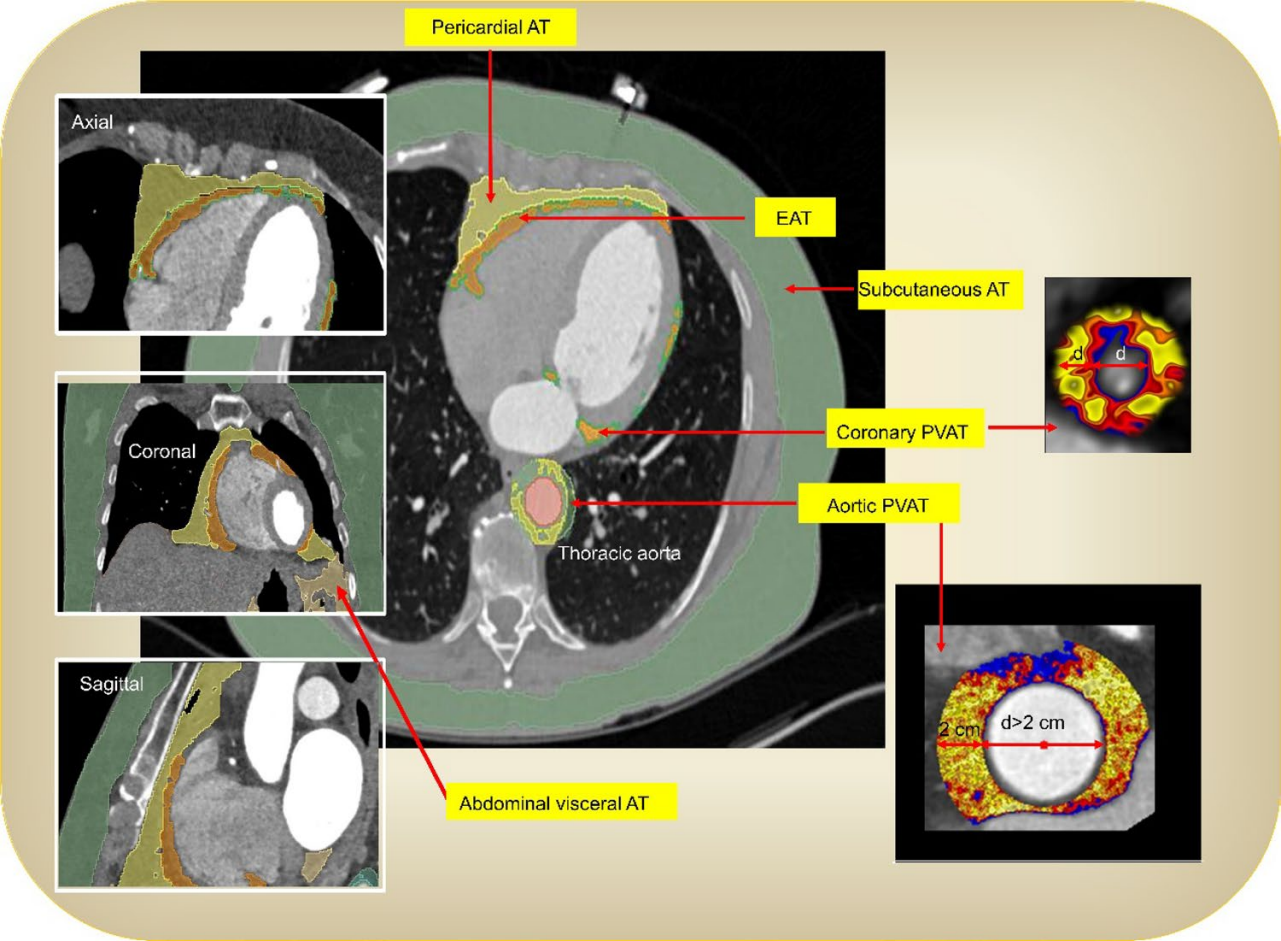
Imaging of human AT depots by CT. This information was modified from Ref [22]
